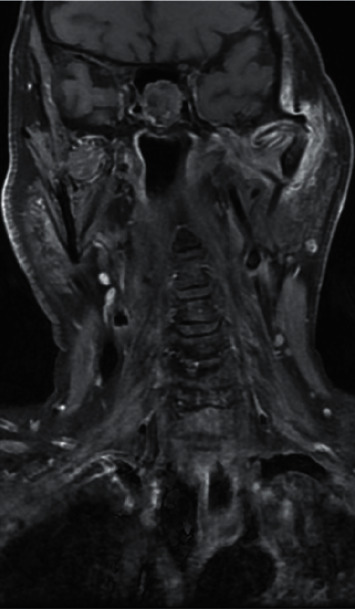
T1-weighted coronal section MRI showing abnormal soft tissue enhancement centred on the left TMJ.
